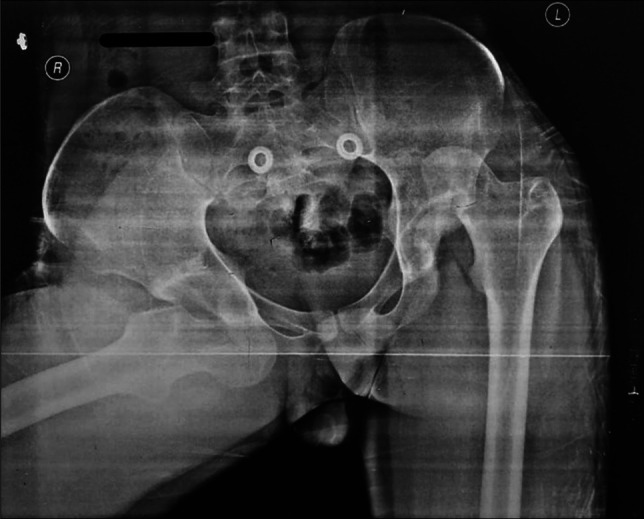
Pre-reduction pelvic radiograph, showing bilateral (right anterior and left posterior) dislocations of the hip.
